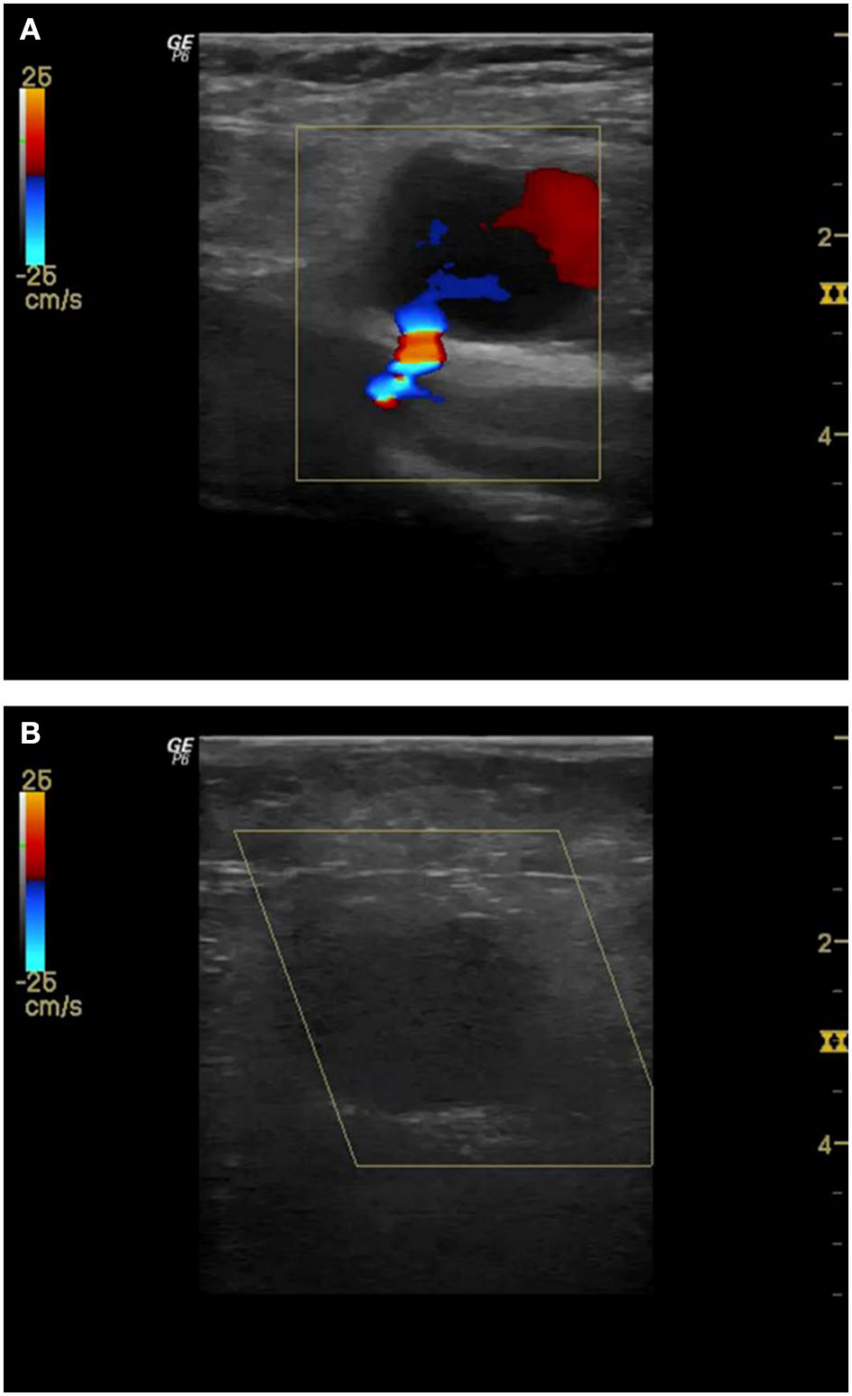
Pseudoaneurysm with clearly visible bleeding from common femoral artery (CFA) after TAVI (A). Since compression with the ultrasound-probe shows no success even after 30 min and an ultrasound-guided injection of 500 IU thrombin is performed. Subsequently, complete closure of the pseudoaneurysm (B).
